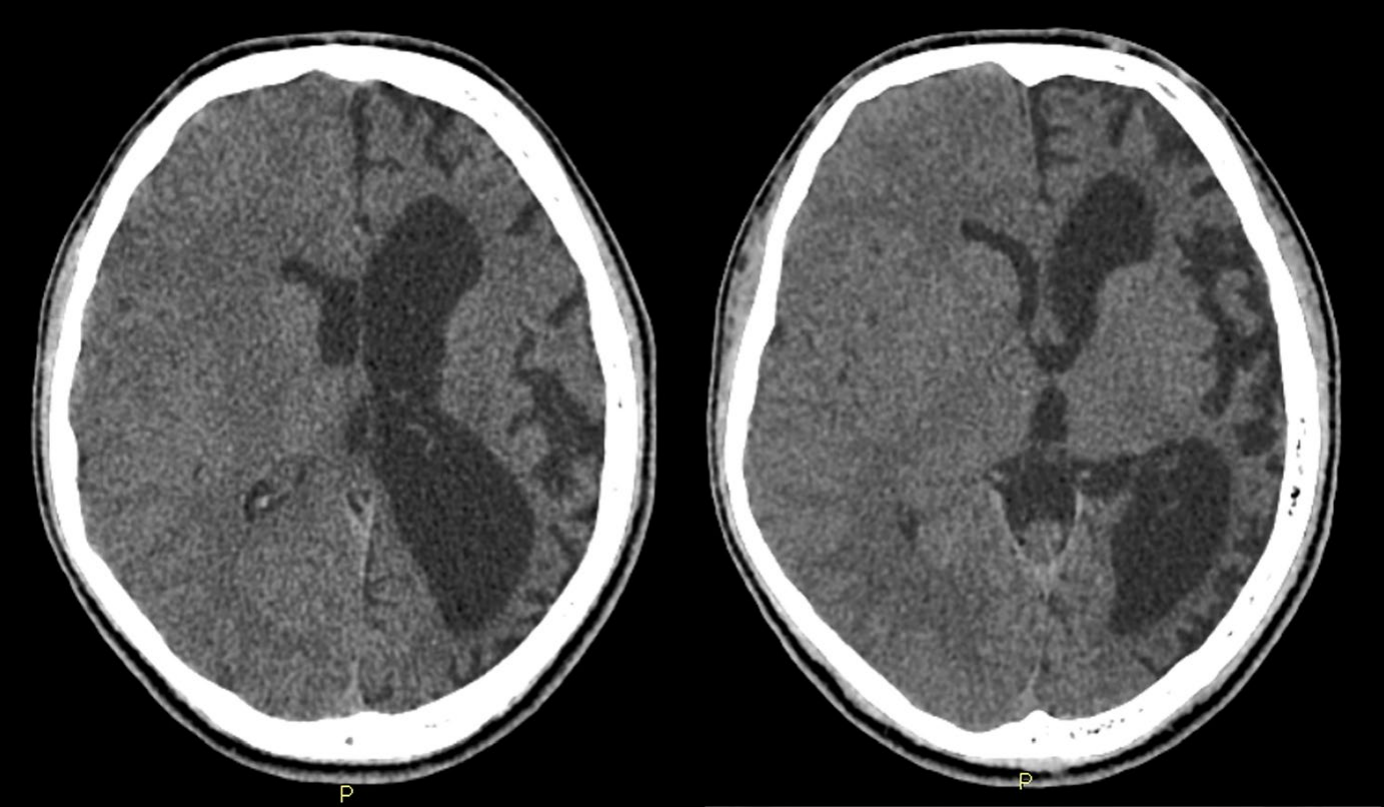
Noncontrast computed tomography of the brain shows atrophy of the left cerebral hemisphere with dilatation of the left lateral ventricle
